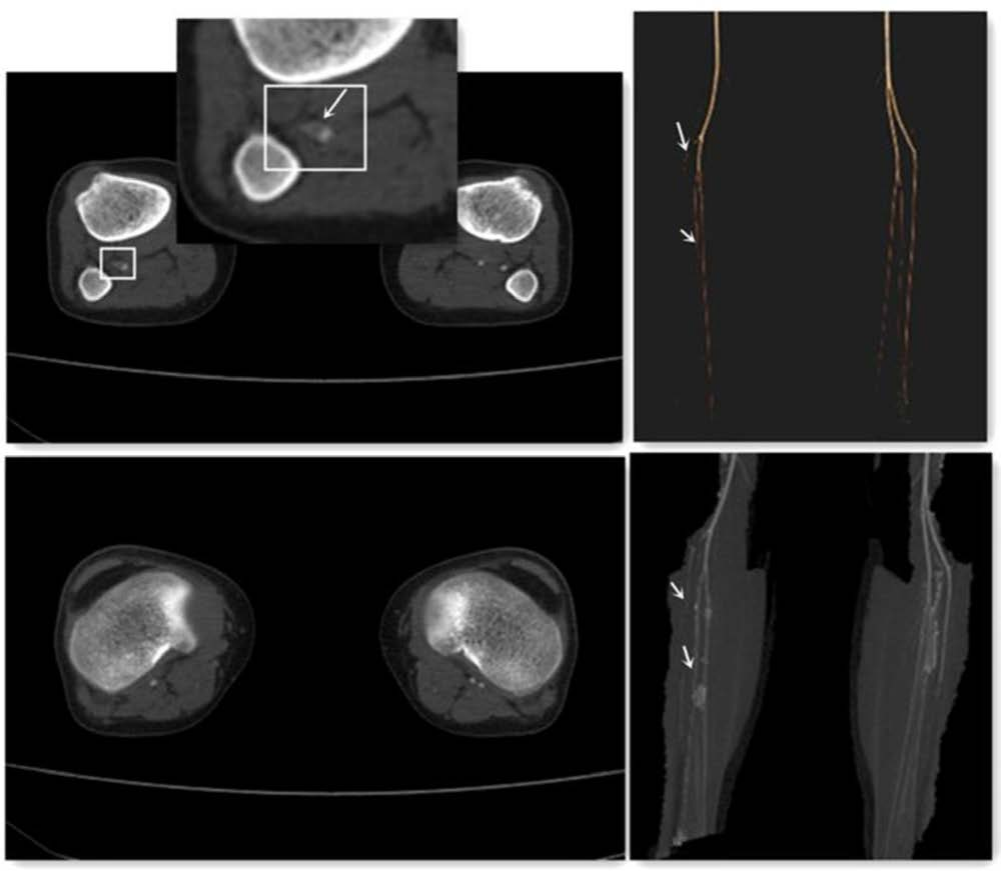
CTA showed that the lumen of the right popliteal artery branch and the beginning of the right anterior tibial artery with filling defects. The right dorsal artery is not visible. CTA = computed tomography angiography.
